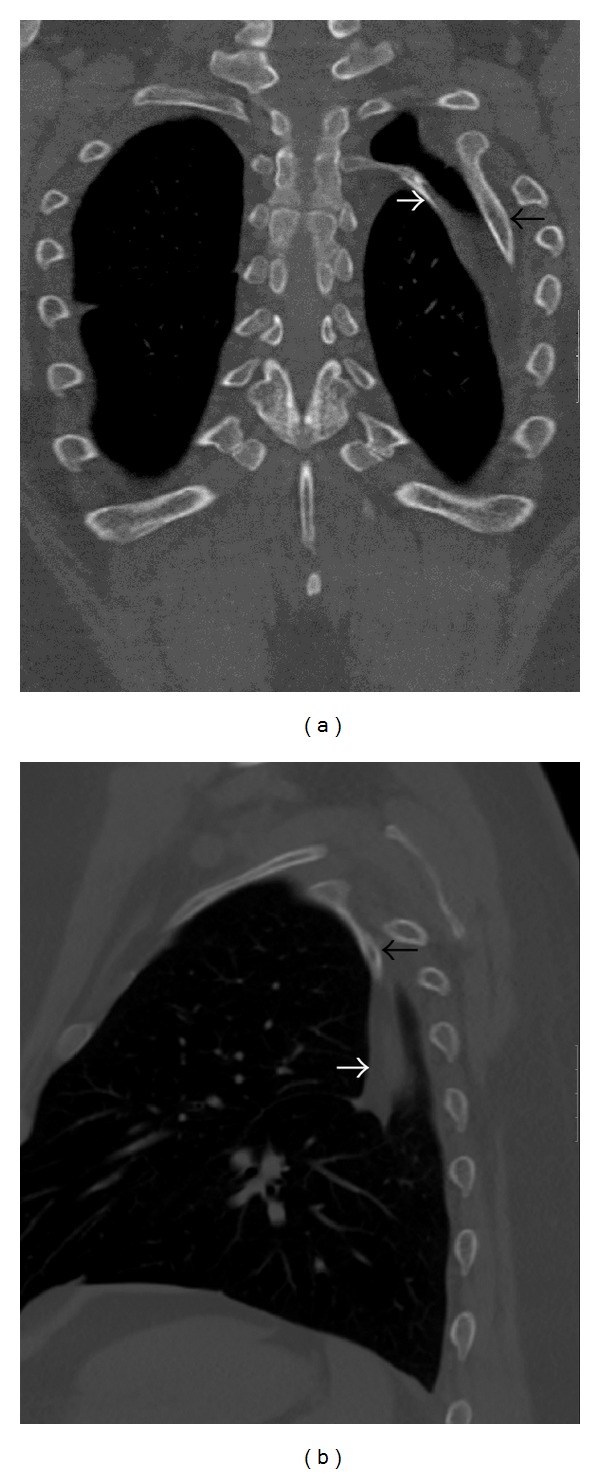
43-year-old gentleman complaining of high grade fever, cough, and chest pain was investigated with chest radiographs and helical chest CT scan and subsequently diagnosed with intrathoracic ribs. Unenhanced chest CT (coronal MIP reformatting, bone window) image in (a) shows left second supernumerary (white arrow) and left third bifid (black arrow) intrathoracic ribs, prominent extrapleural soft tissue around these ribs, and hypoplastic left lung. Unenhanced chest CT (sagittal MIP reformatting, bone window) image in (b) shows left third bifid intrathoracic rib (black arrow) and prominent extrapleural soft tissue around it (white arrow).
